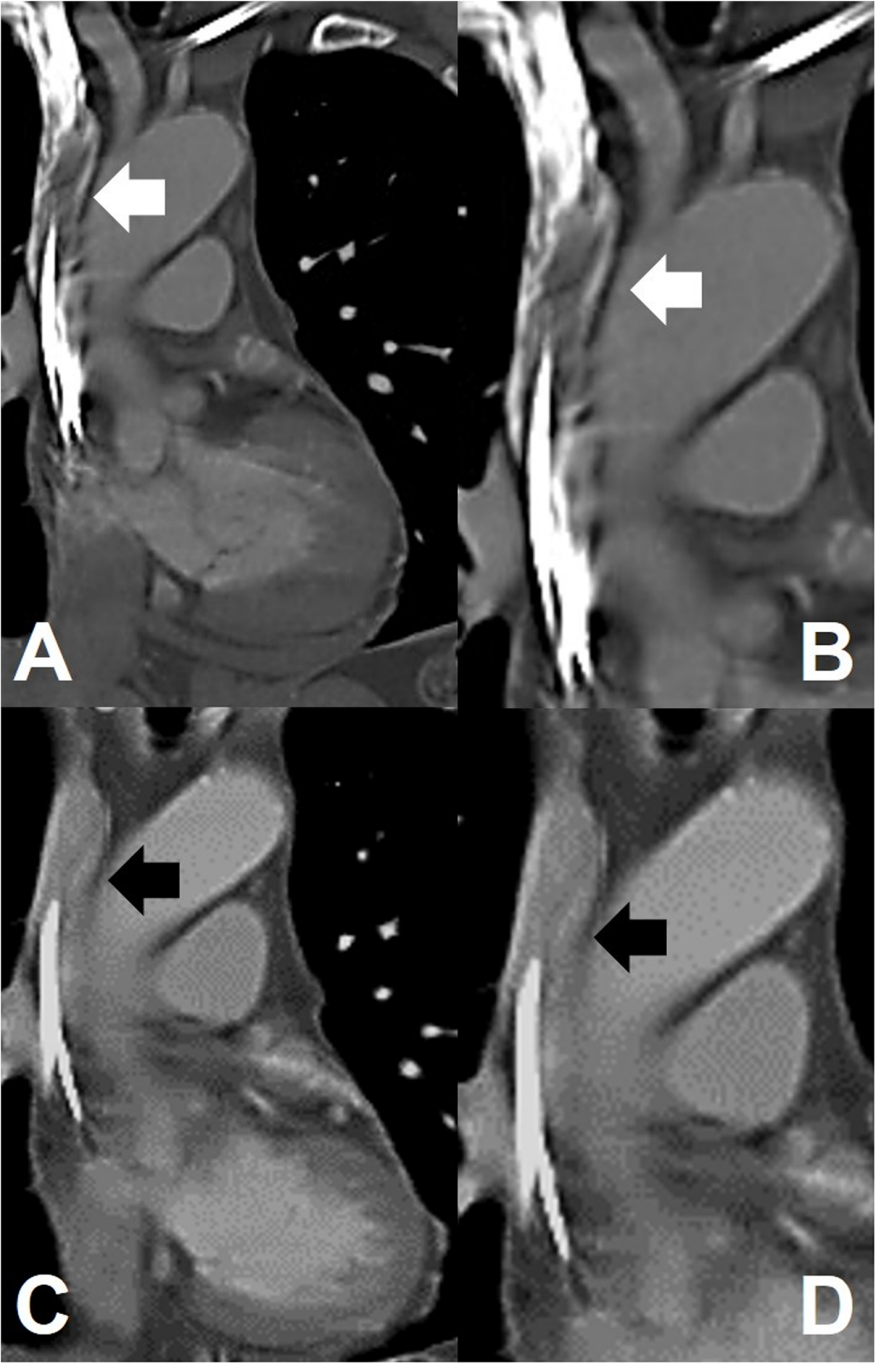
Chest computed tomography. A, B. A filling defect in the right internal jugular vein and superior vena cava was noted with highly suspected lead thrombosis coated on the pacemaker leads (white arrows). Follow-up chest computed tomography. C, D. No filling defect and no residual lead thrombus were noted (black arrows)
